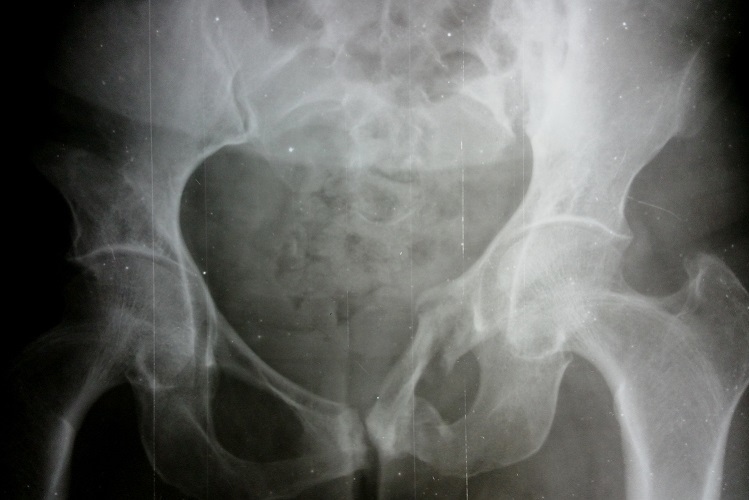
Radiographie du bassin (face), ostéolyse de la branche ilio-pubienne gauche
